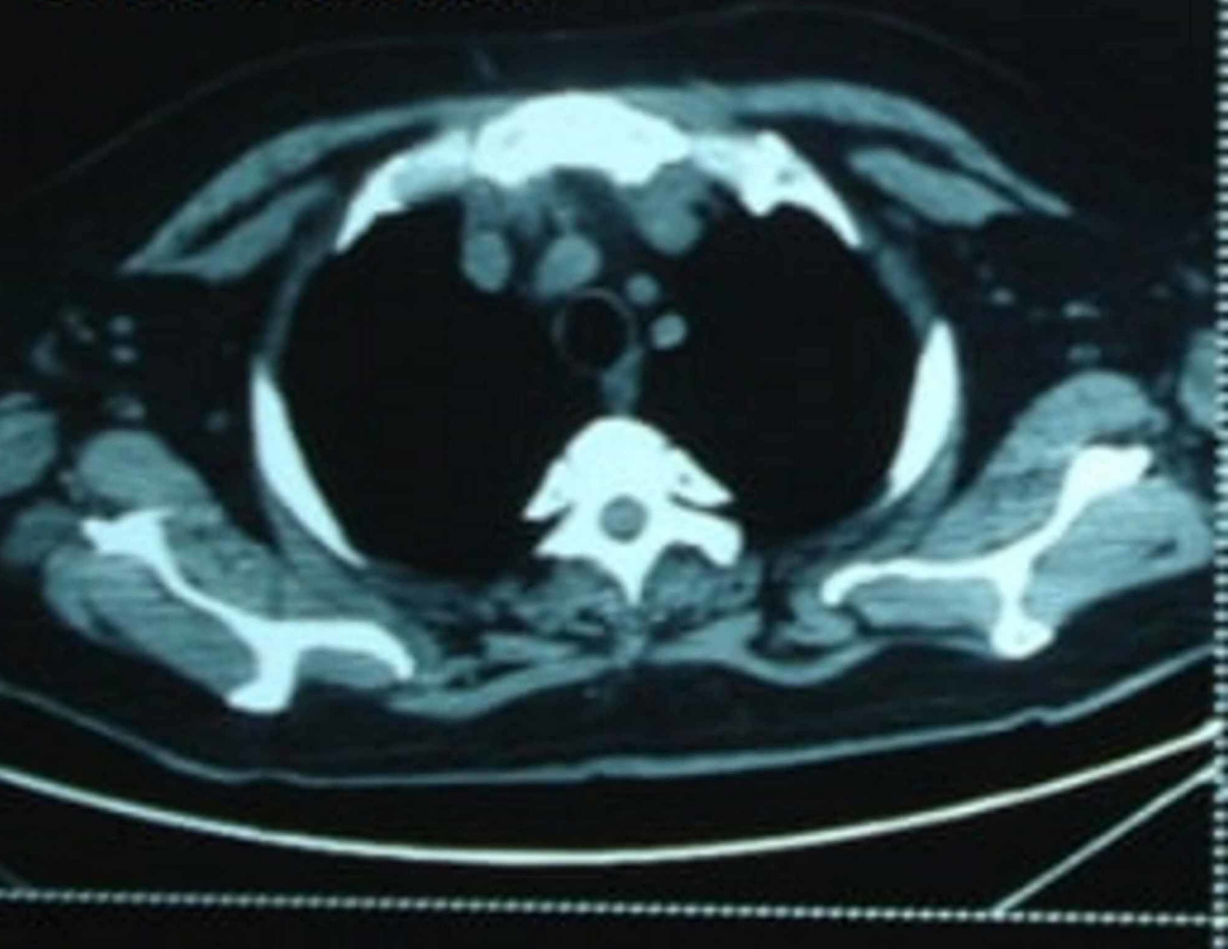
Computed tomography (CT) scan six months later showing resolution of the mediastinal abscess
